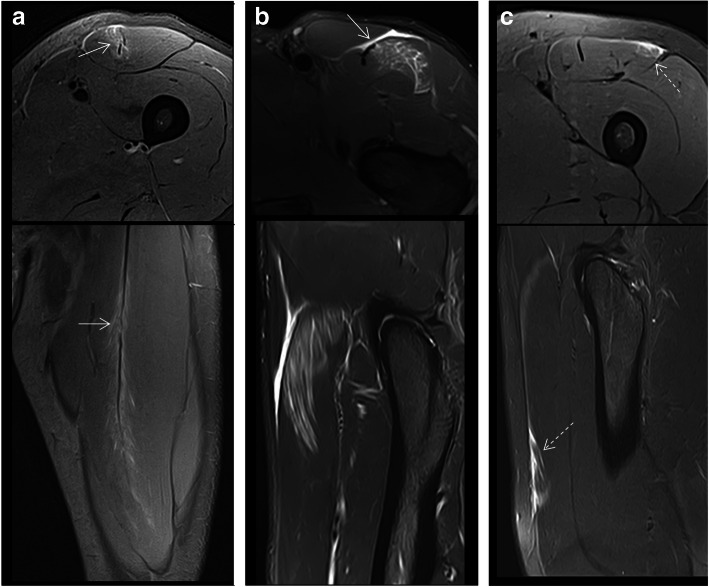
Proximal lesions of the rectus femoris. Fluid-sensitive MRI sequences showed (a) gap of the central septum with edema and loss of the pennation angle of the muscle fibers of myoconnective anchor center (axial and coronal images); gap of the aponeurosis (arrows) with interfascicular and intermuscular hemorrhage (b) (axial and sagittal images); discontinuity of the connective tissue of lesser thickness (dashed arrows) with peripheral fluid and fiber gap in the anchorage of peripheral myoconnective fibers (c) (axial and sagittal images)
